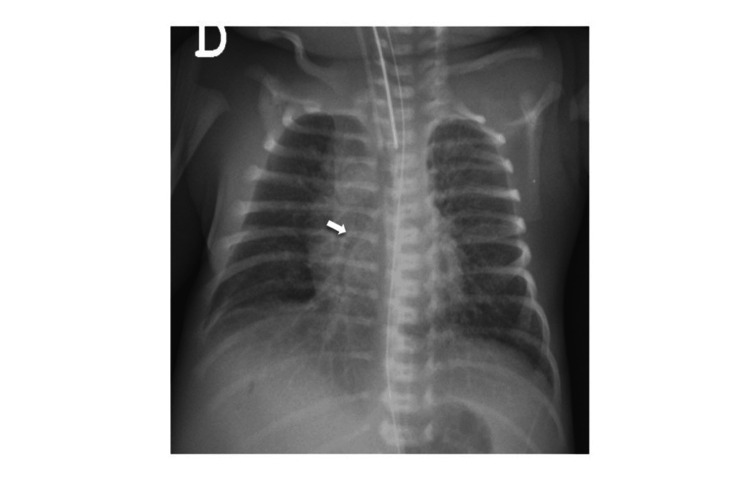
Chest radiograph, anteroposterior incidence, showing a diffuse granular pattern, with air bronchogram (white arrow), and no signs of pleural effusion or pneumothorax.
